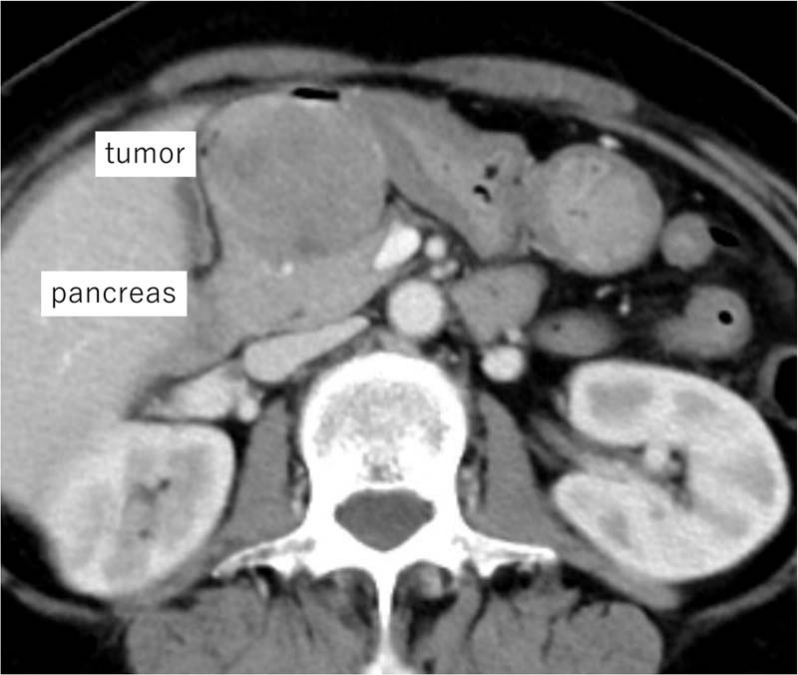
Imaging of the tumor. Contrast-enhanced CT showing a 5 cm large mass with contrast effect on the posterior wall of the duodenal bulb and pancreatic head invasion.
